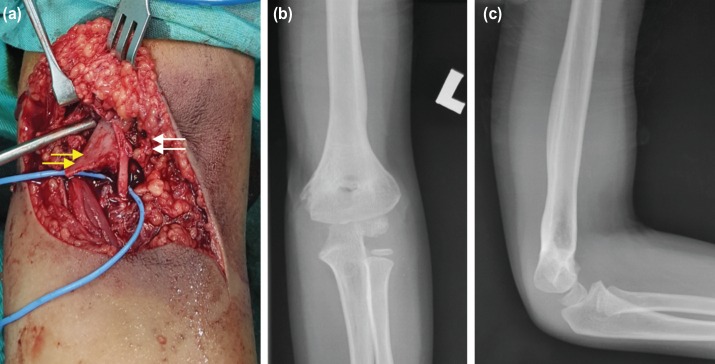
(a) Intra-operatively, the brachial artery is partially lacerated (white arrow) by the sharp edge of the metaphyseal spike (yellow allow). The median nerve appears intact. (b, c) Radiographs of the left elbow (frontal and lateral projections) taken during a final assessment at one year post-operatively showed a well-united fracture.
